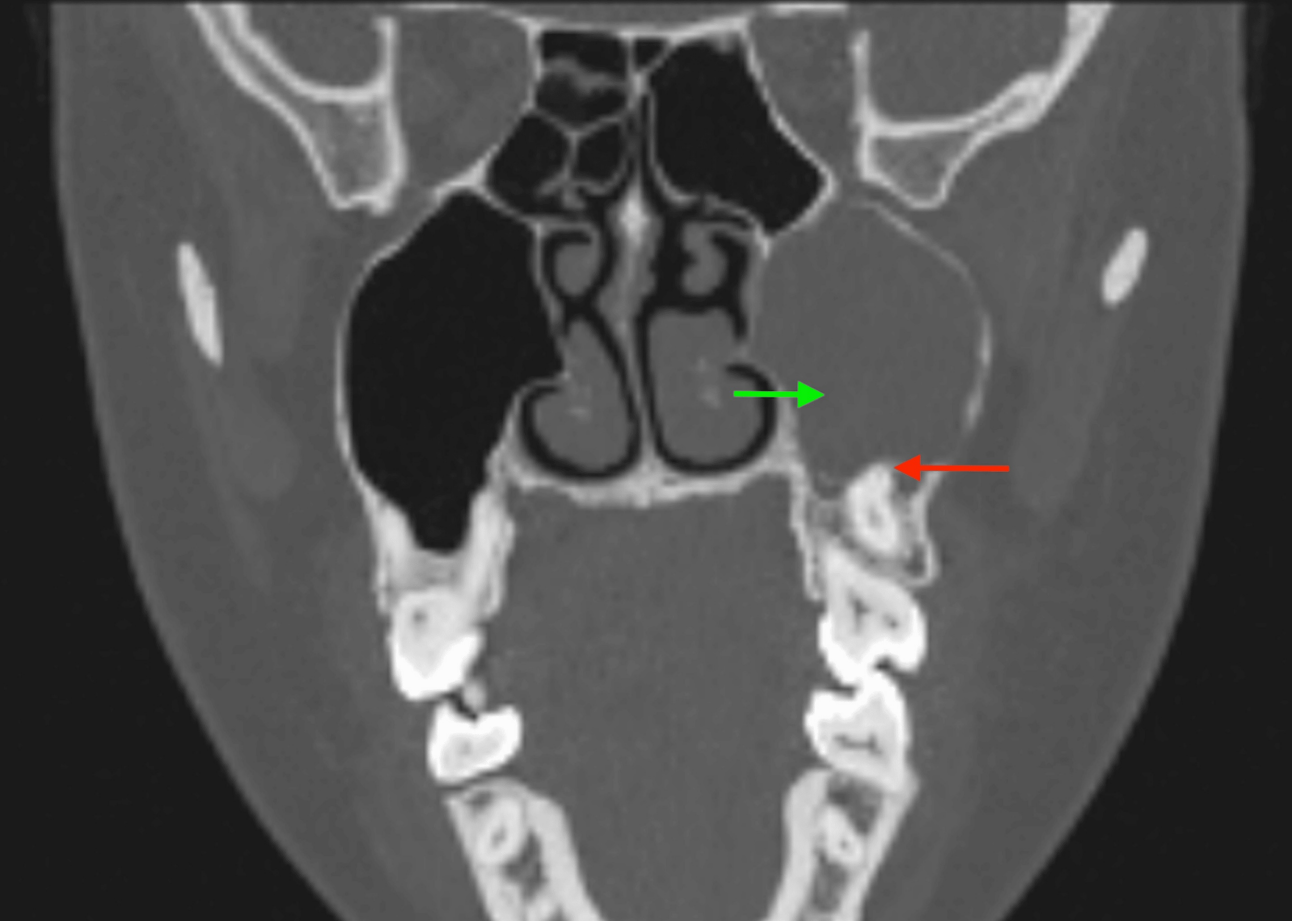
Coronal computed tomography scan showing the pericoronal cyst (green arrow) with an ectopic tooth (red arrow).
